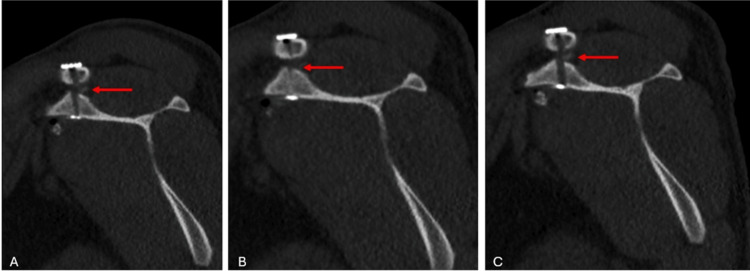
Postoperative sagittal CT images of the patient The images show the tunnel placement in the coracoid bone and clavicle, confirming proper implant positioning within the tunnel. The red arrows indicate the tunnel.
